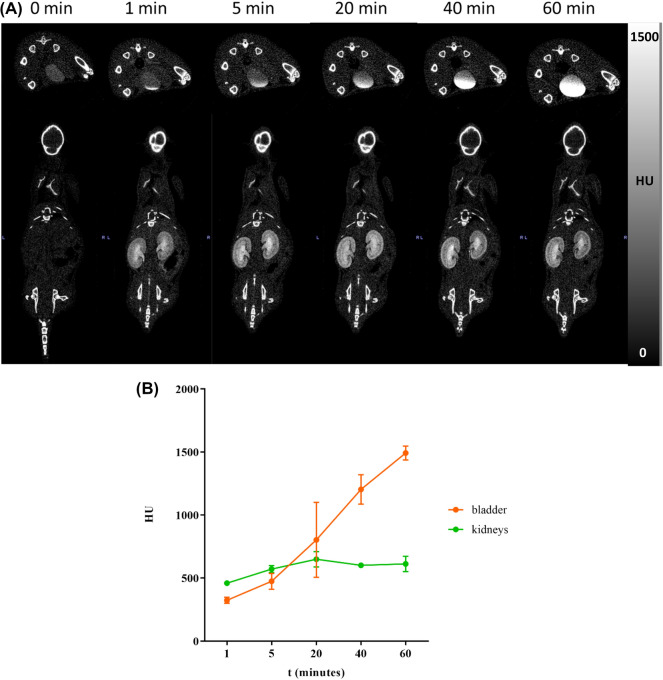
(A) In vivo CT imaging after *i.v.* administration of 5 mmol/kg of Bi-HPDO3A. Bladder and kidneys CT contrast are displayed. (B) VOIs analysis on bladder and kidneys for a dose of 5 mmol/kg of Bi-HPDO3A.
